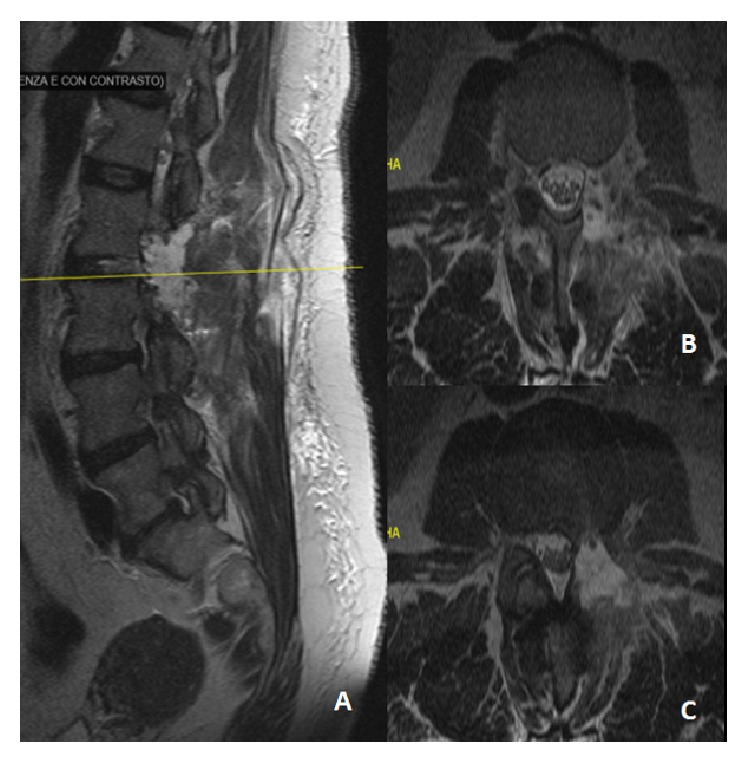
A postoperative MRI scan demonstrating complete resection of the sequestered disc material. Note the decompression of the dural sac and nerve root. L2-L3 arthrectomy and interspinous fixation at the same level were performed.
